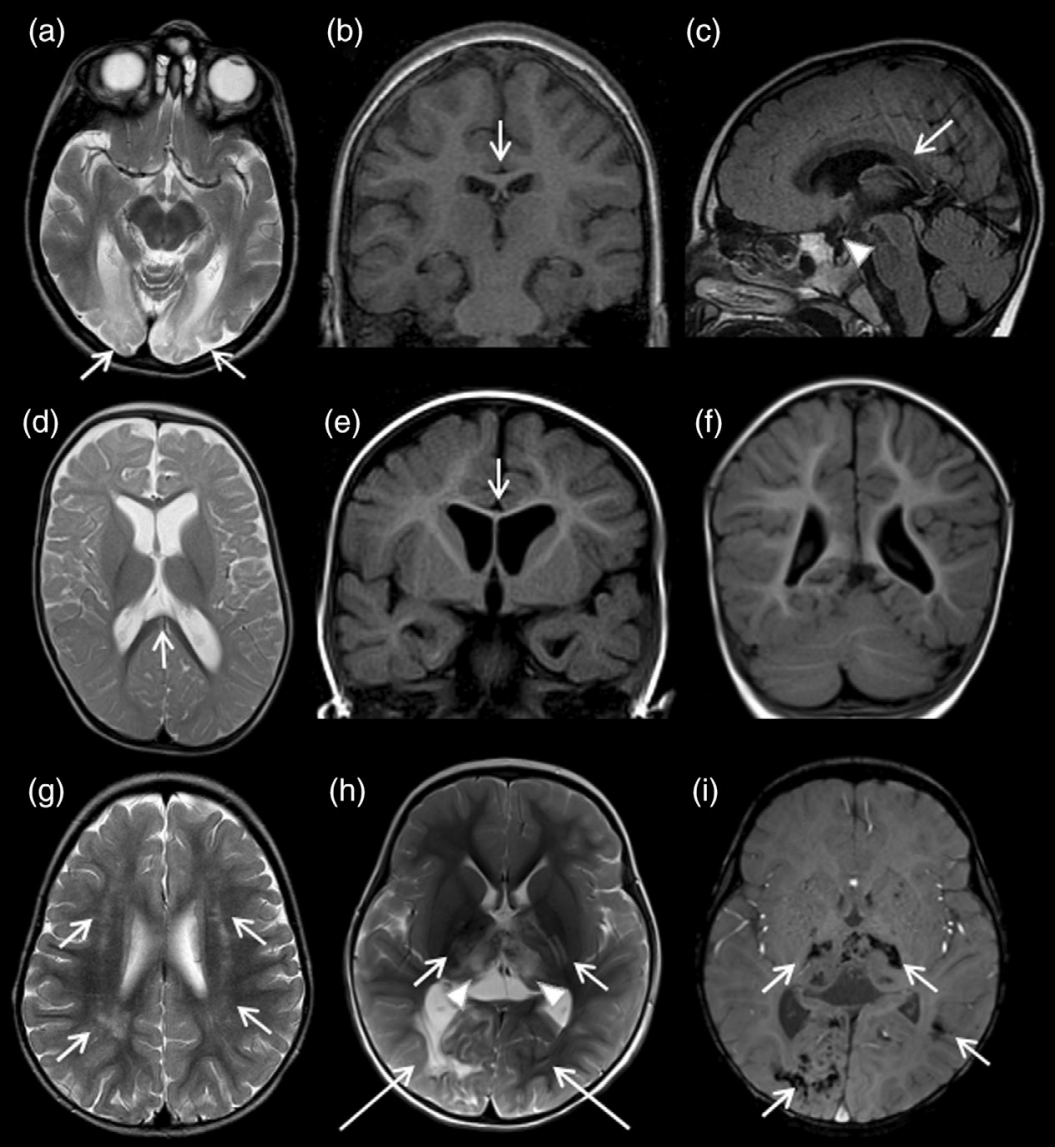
Brain magnetic resonance imaging (MRI) patterns of the uniquely prevalent genetic diseases of this Northern Finnish cohort. Two different patients with seizures, cortical blindness, and microcephaly syndrome at the age of 8 years (a and b) and 4 years (c). Axial T2‐weighted image shows bilateral and symmetrical signal abnormalities, and atrophy in occipital lobes (arrows in a). The corpus callosum shows mild thinning in both patients (arrows in b and c). The optic chiasm is markedly thin (arrowhead in c). Axial T2‐weighted image (d) and coronal T1‐weighted images (e and f) of a 10‐month‐old patient with fibrosis, infection susceptibility/immunodeficiency/intellectual disability, neurodevelopmental disorder/neurodegeneration and chronic anemia/cerebral angiomatosis disease shows thinning of the corpus callosum (arrows in d and e) and marked cerebral cortical atrophy (d–f). A patient with ataxia‐pancytopenia syndrome at the age of 1 year 3 months presents multifocal white matter signal abnormalities in the frontal and parietal lobes on axial T2‐weighted image (arrows in g). A patient with cerebroretinal microangiopathy with calcifications and cysts at the age of 2 years 2 months demonstrates bilateral signal abnormalities in the thalami (arrowheads in h), internal capsule (short arrows in h), and occipital lobes (long arrows in h) on axial T2‐weighted image and marked calcifications in the affected areas on susceptibility weighted imaging (arrows in i).
